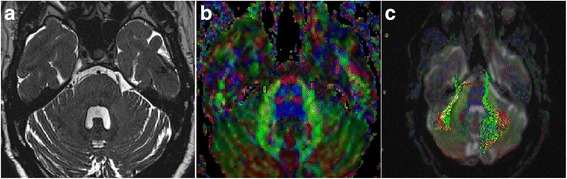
Pre-operative MRI of patient diagnosed as TN. a Routine MRI sequences displayed the nerve and vascular conflicting on the right side. b Diffusion tensor images and the region of interest measured of bilateral TGN. c The reconstruction of TGN fibers is shown
